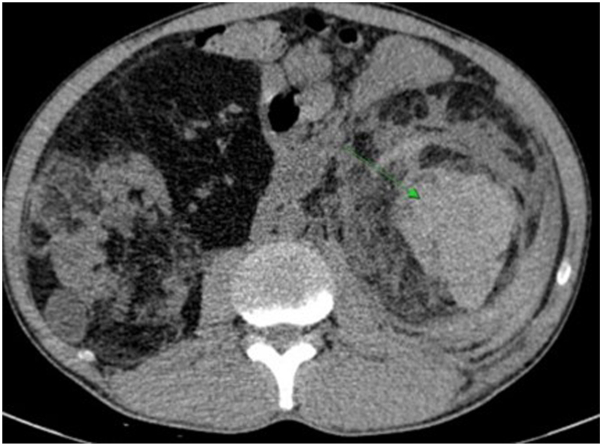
Contrast-enhanced CT of the abdomen showing a ruptured left renal angiomyolipoma (*arrow*) with a large retroperitoneal hematoma. Multiple bilateral renal AMLs are also visible.
